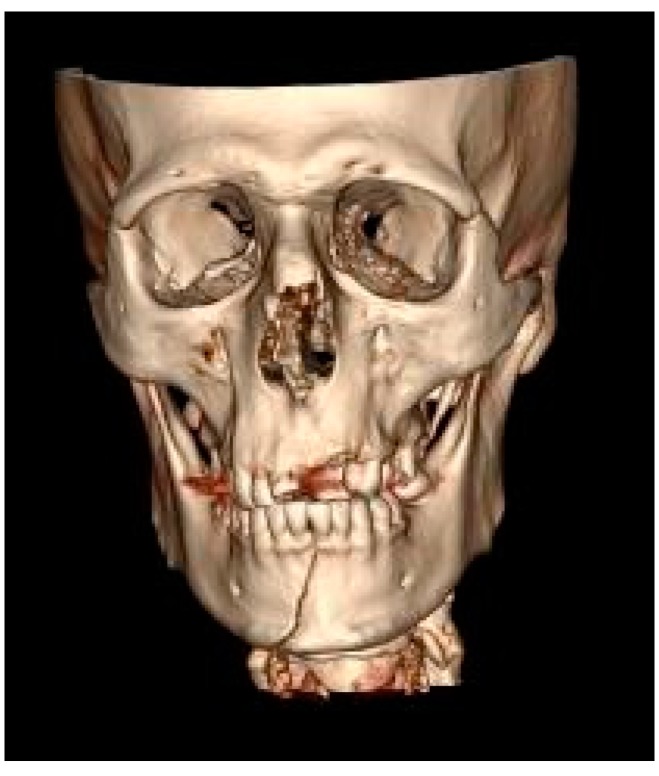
Mandibular symphyseal fracture (computed tomography with 3D reconstruction).
